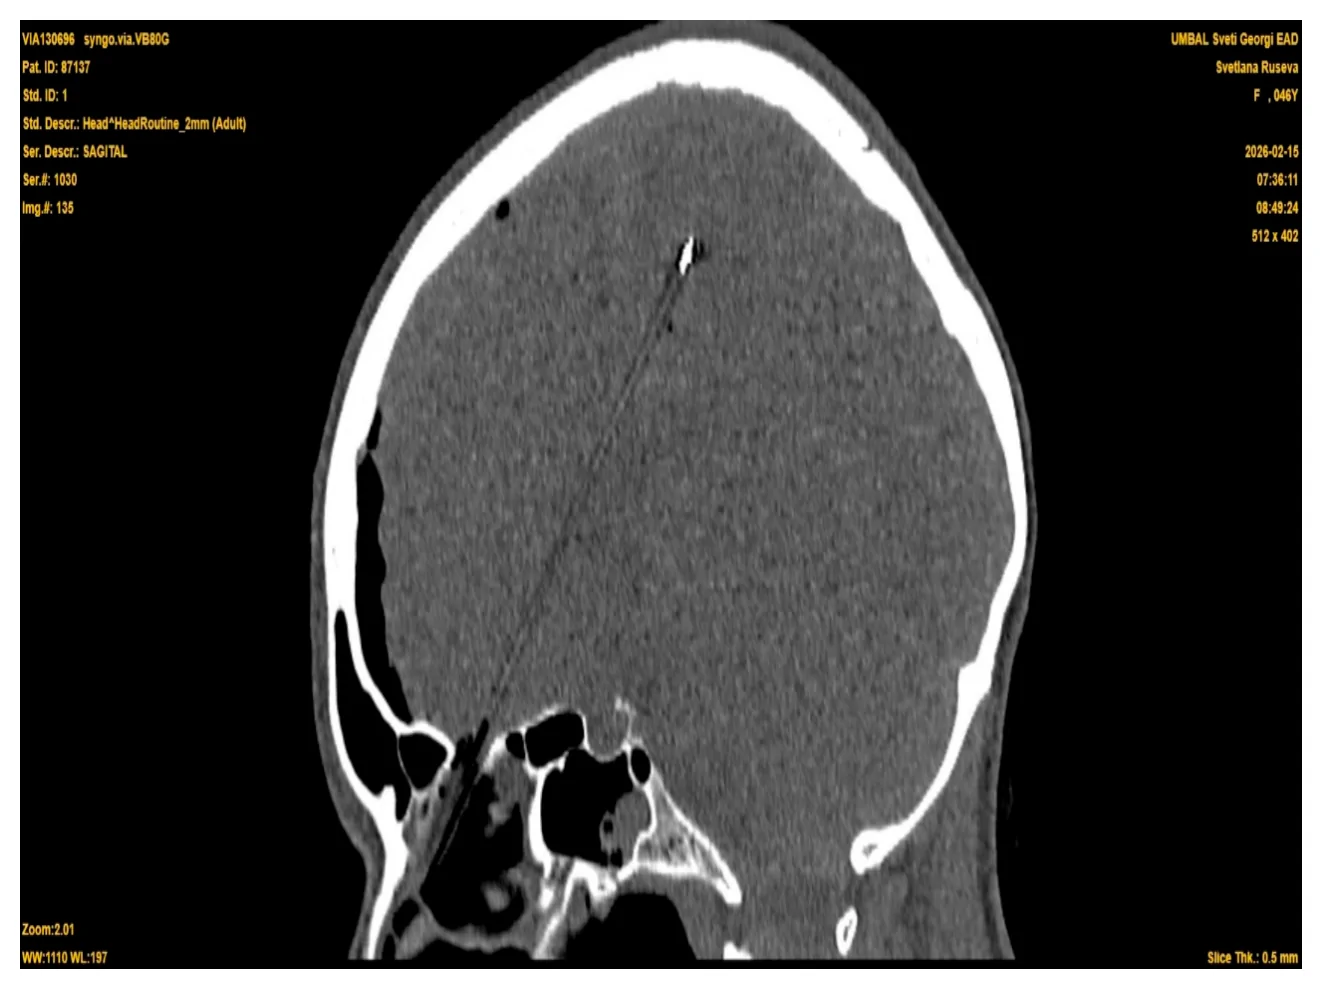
Preoperative CT scans demonstrating the localization of the intracranial foreign body.
